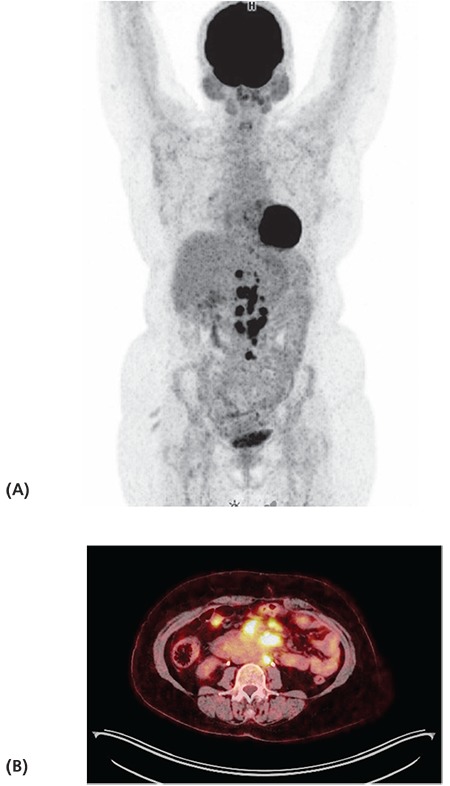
Maximum intensity projection (A) and axial fused positron emission tomography/computed tomography (B) images of a 49 yearold patient with serous carcinoma (patient no: 26) show increased ^18^F-FDG uptake in para-aortic and celiac lymph nodes (SUV_max_: 18.3) and mesenteric implants (4.2). Peritoneal biopsy confirmed malignancy in this patient
